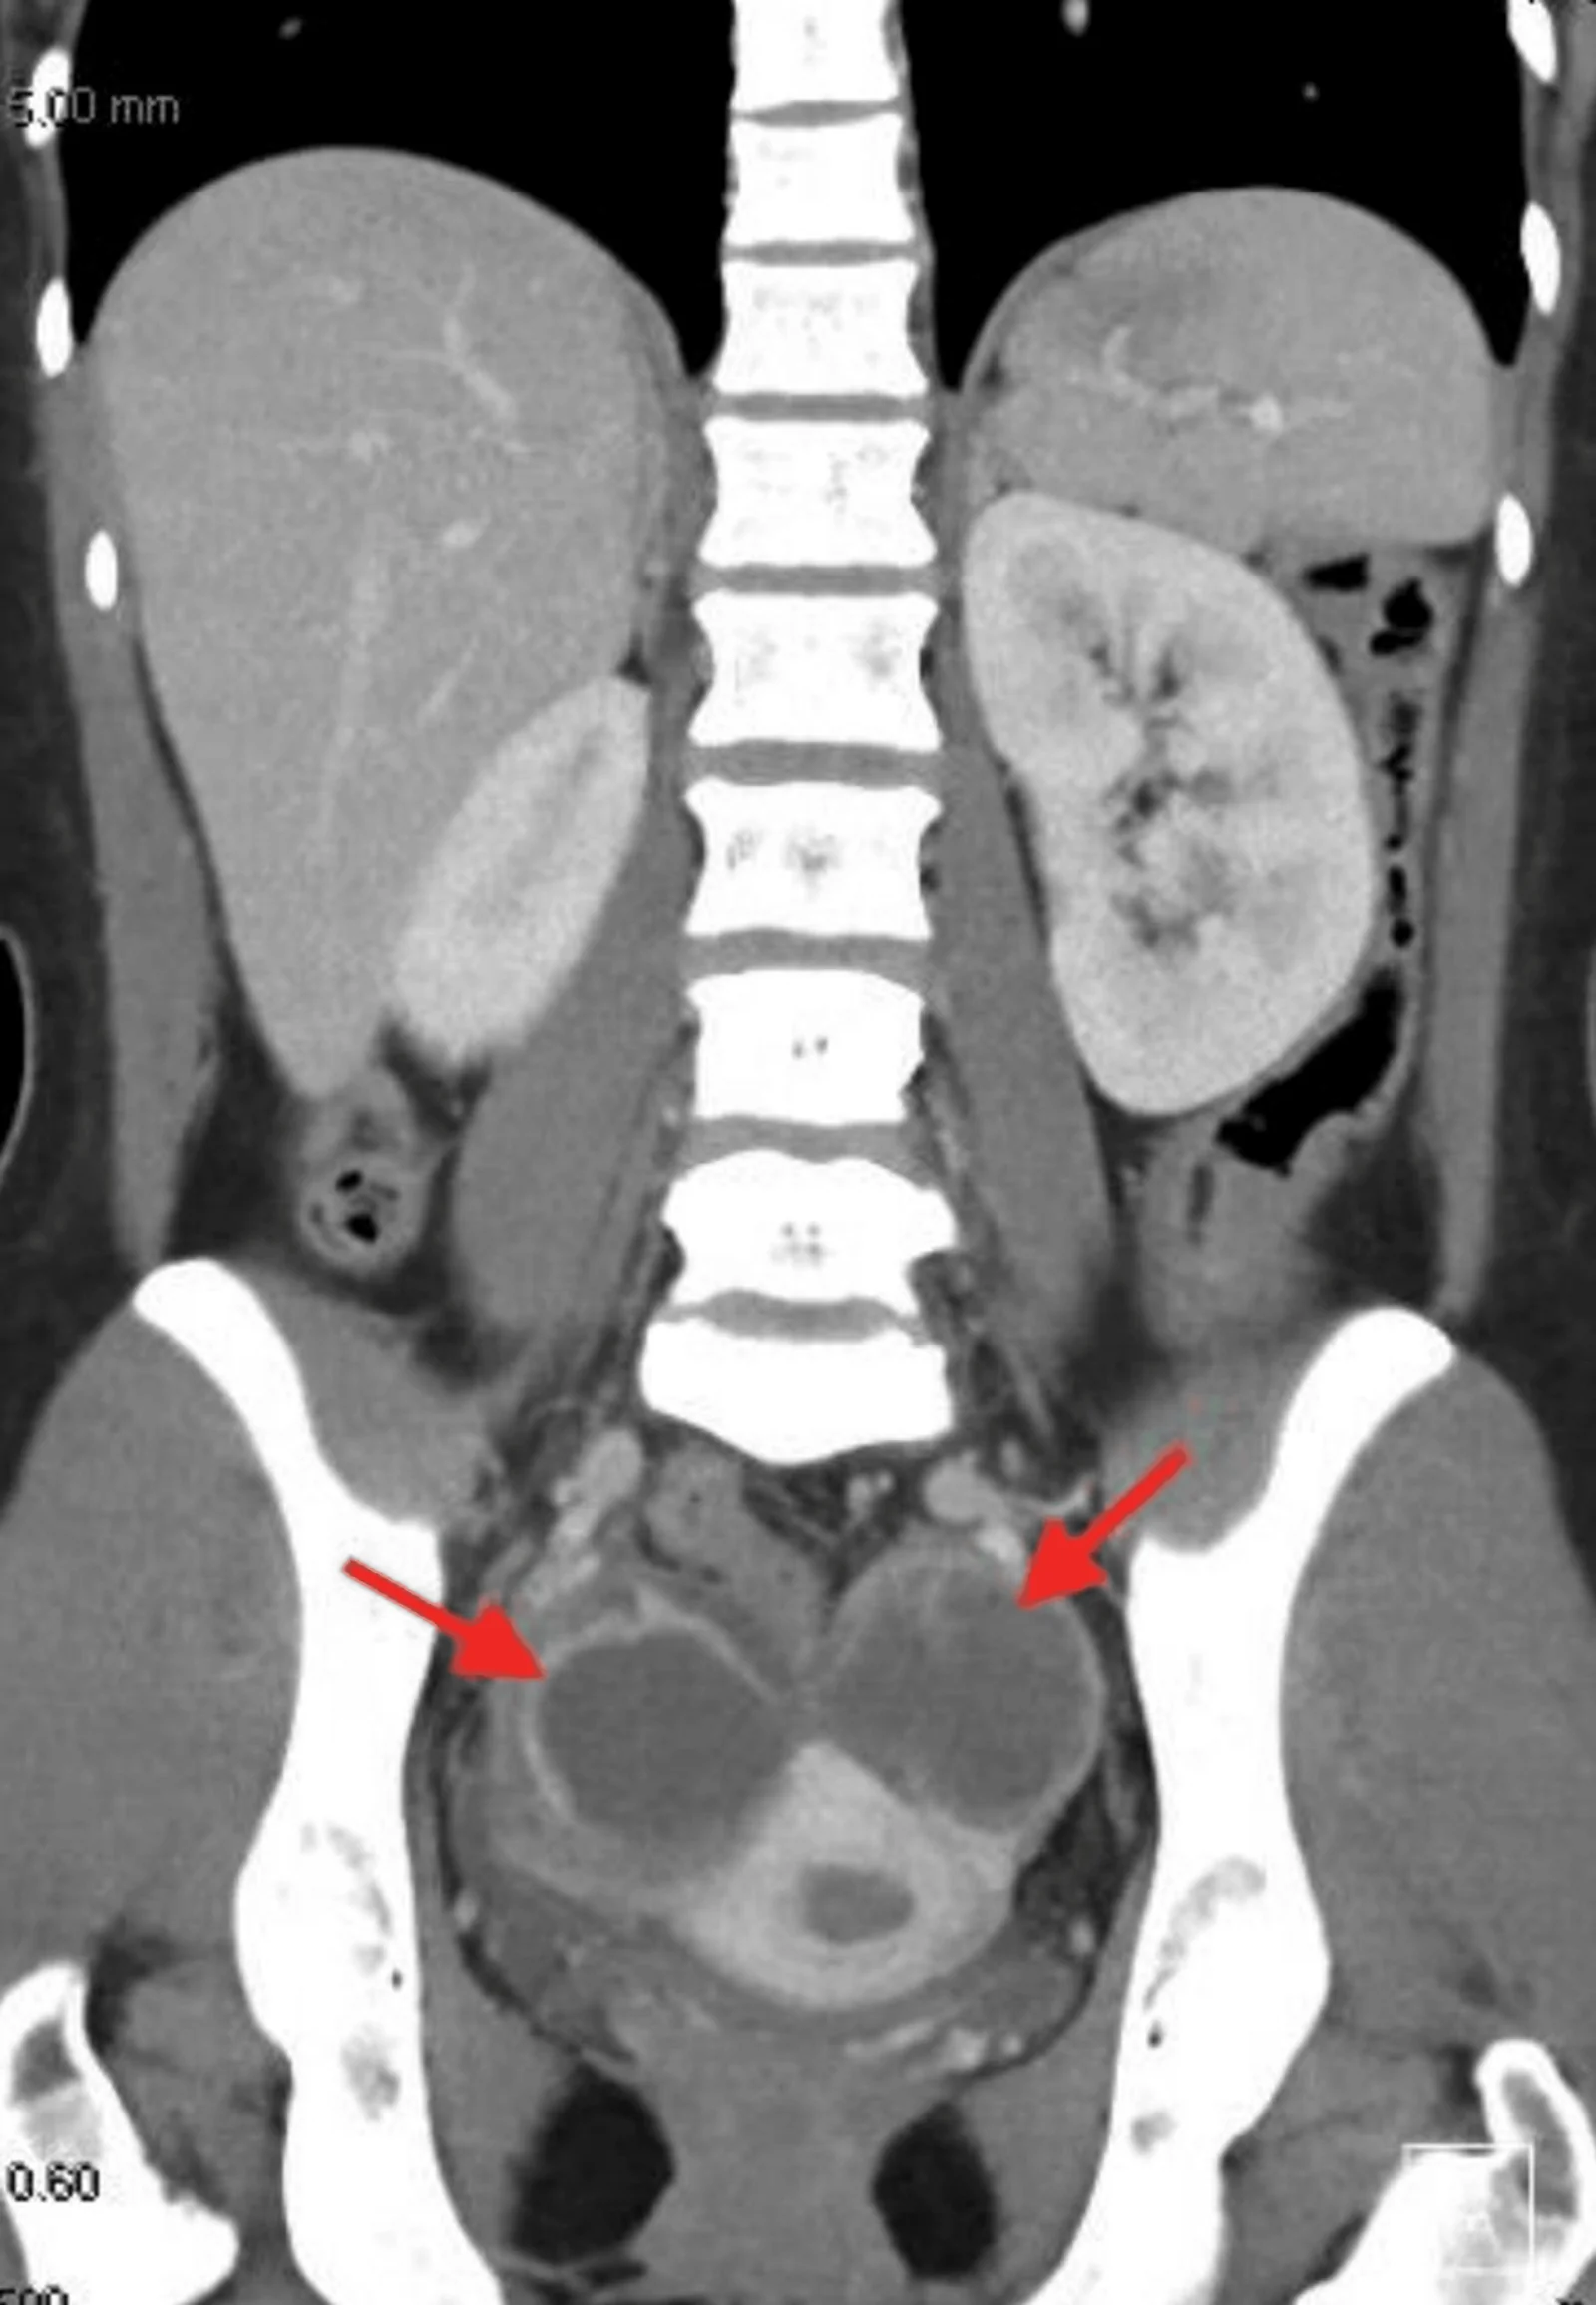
Coronal contrast-enhanced CT showing bilateral adnexal lesions Cystic and solid multiloculated bilateral adnexal lesions (red arrows), measuring up to 7.6 × 7.8 cm on the right. Correlation with pelvic ultrasound is recommended. CT: computed tomography
